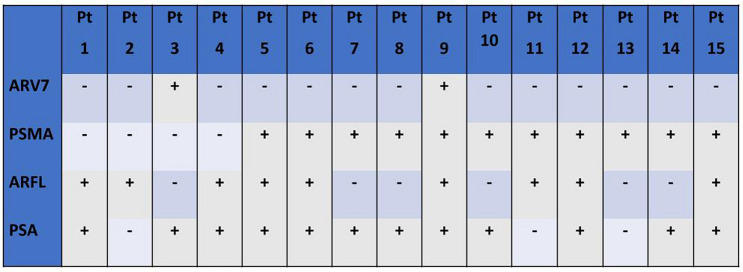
Expression of ARV7, PSMA, ARFL and PSA in all CTC positive patients (pt 1–15)
